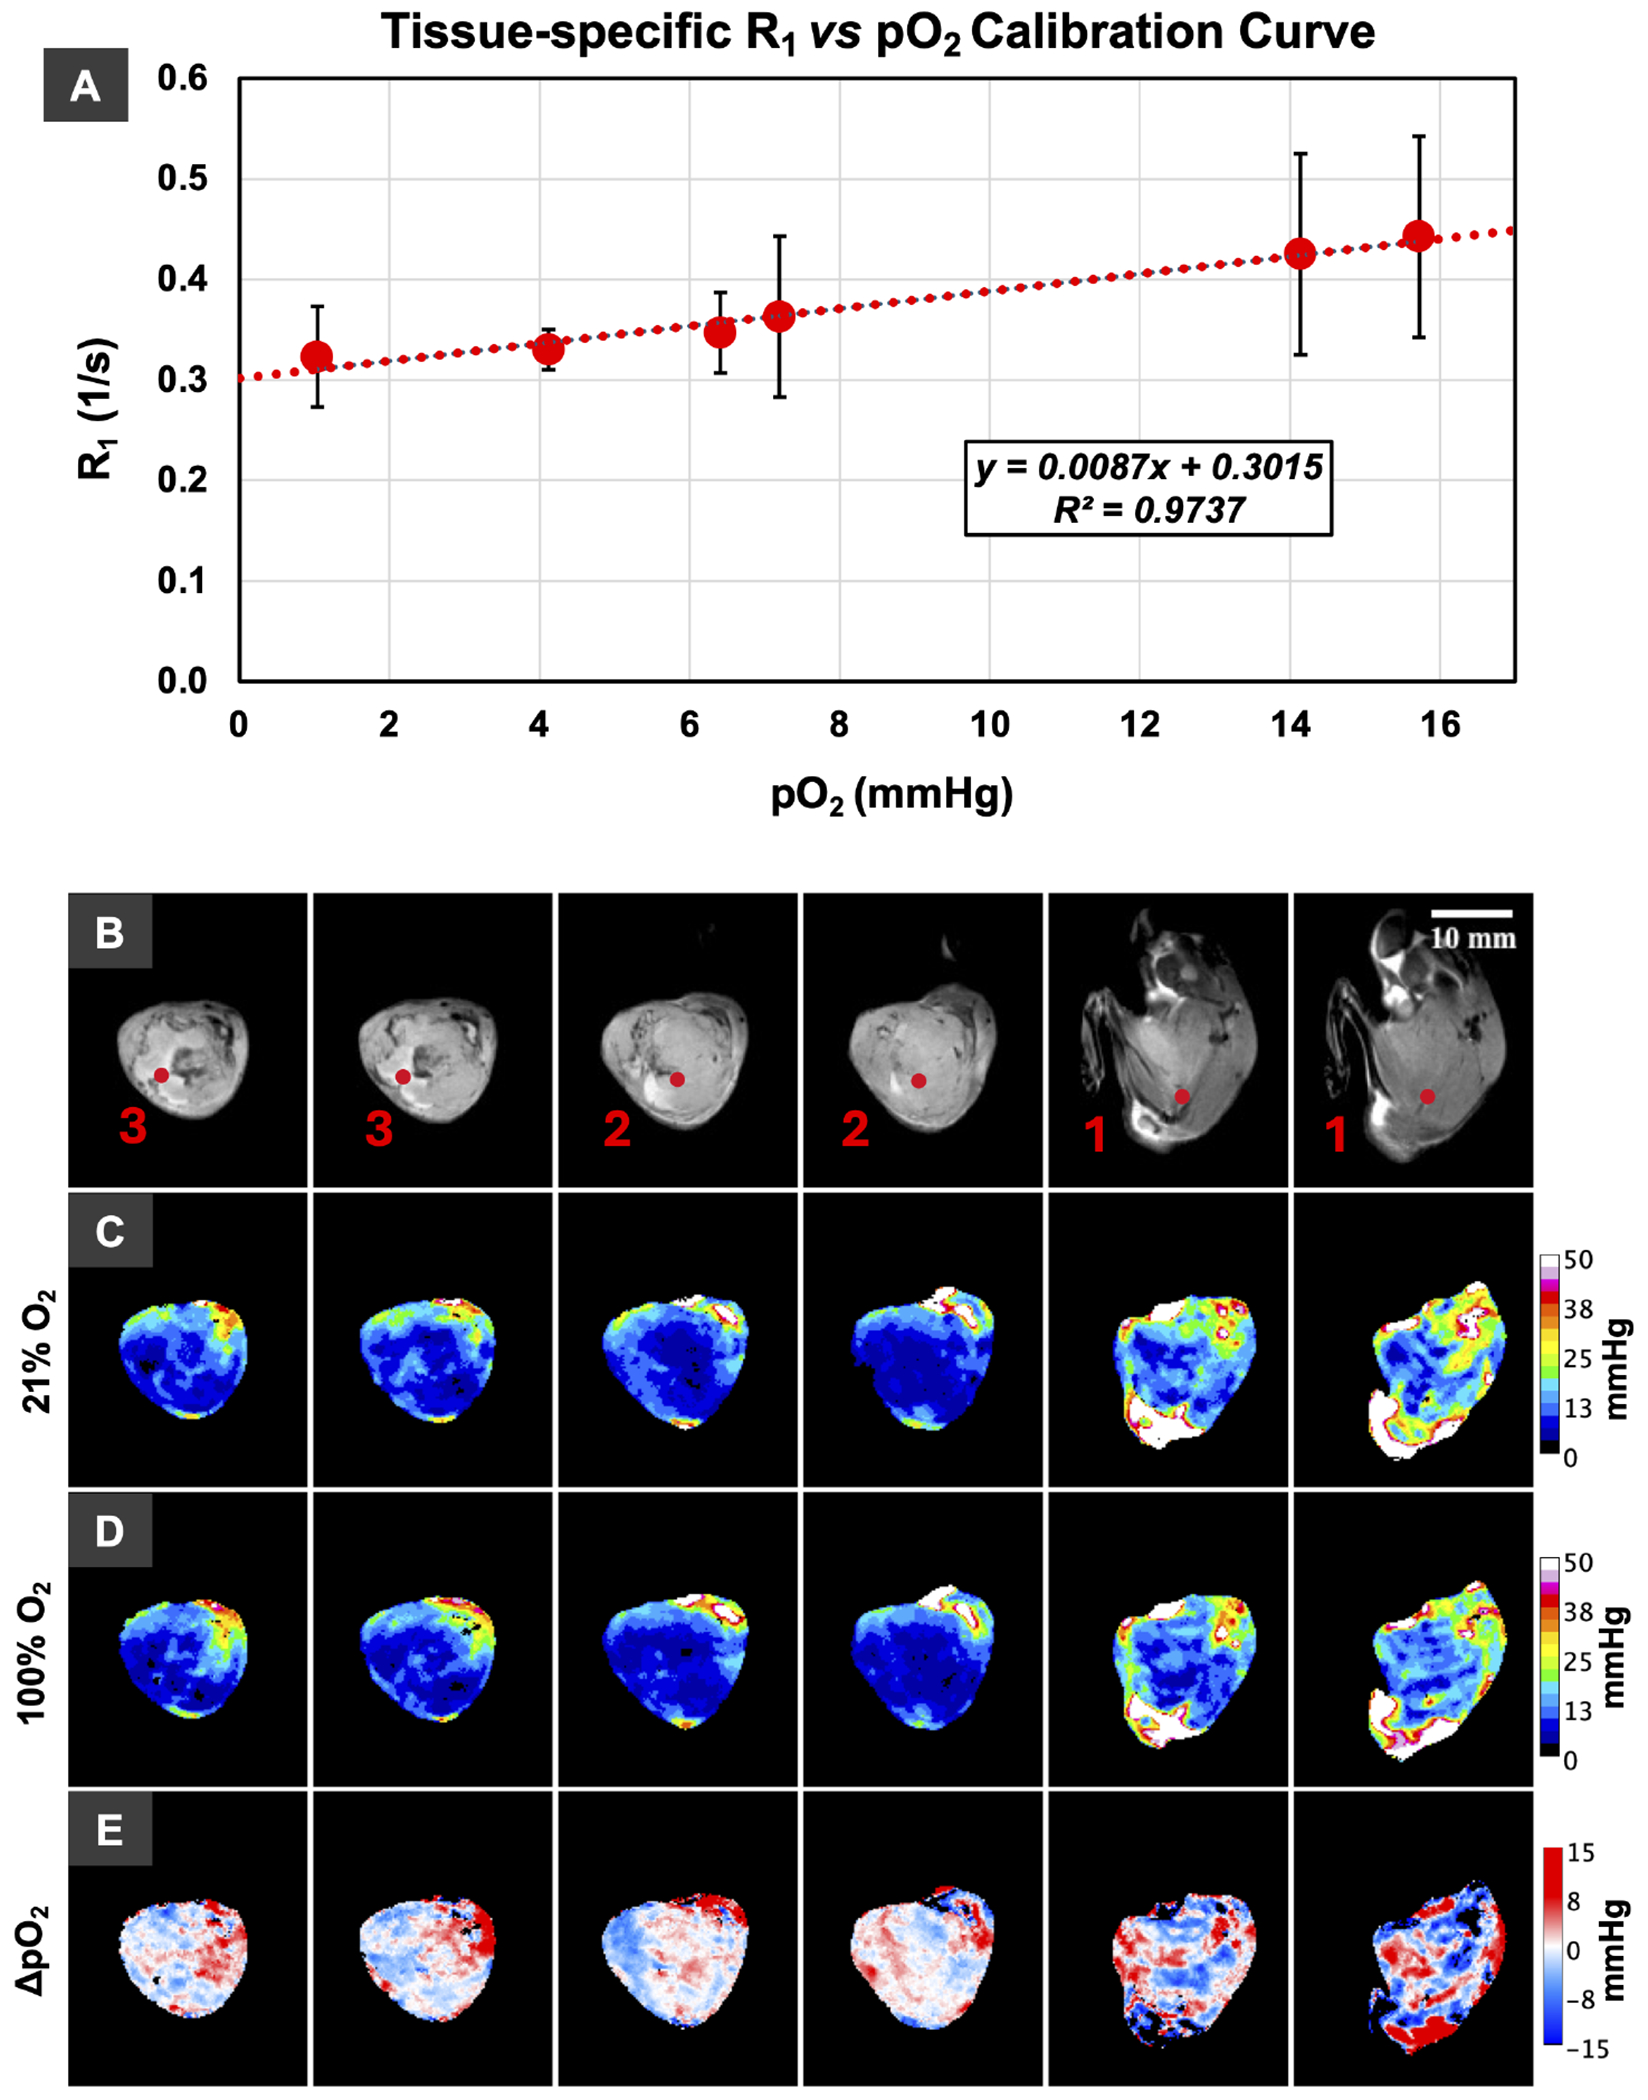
qOE-MRI results. (A) Tissue-specific R_1_ –pO_2_ linear calibration curve. (B) T_1_-weighted anatomical scan of the tumor and surrounding leg muscle showing the slices where the OxyChips are visible due to the slight susceptibility artifacts caused by the GNPs. Each OxyChip is demonstrated in two slices — same OxyChip labeling convention as in Figures 4 and 5. An R_1_ map is generated by taking the inverse of the T_1_map. The average R_1_ value of the tissue nearest to the OxyChip (within 300 μm) is recorded and averaged between slices. A calibration curve is generated by correlating the recorded R_1_ values and the measured pO_2_ values from EPR oximetry for each breathing condition. (C) pO_2_ map of the tumor and surrounding leg muscle at 21% O_2_. (D) pO_2_ map of the tumor and surrounding leg muscle at 100% O_2_. (E) Delta pO_2_ between 100% and 21% O_2_ breathing conditions. Red-colored numbers indicate OxyChip positions.
